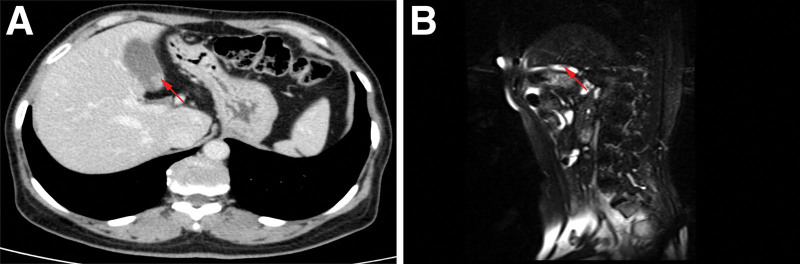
Contrast-enhanced CT (A) of the gallbladder shows irregular nodular enhancement foci in the neck of the gallbladder, measuring approximately 1.2 × 0.9 cm. A long striated lesion is seen in the sagittal position of T2WI of the gallbladder(B). The red arrow points to the lesion. CT = computed tomography.
